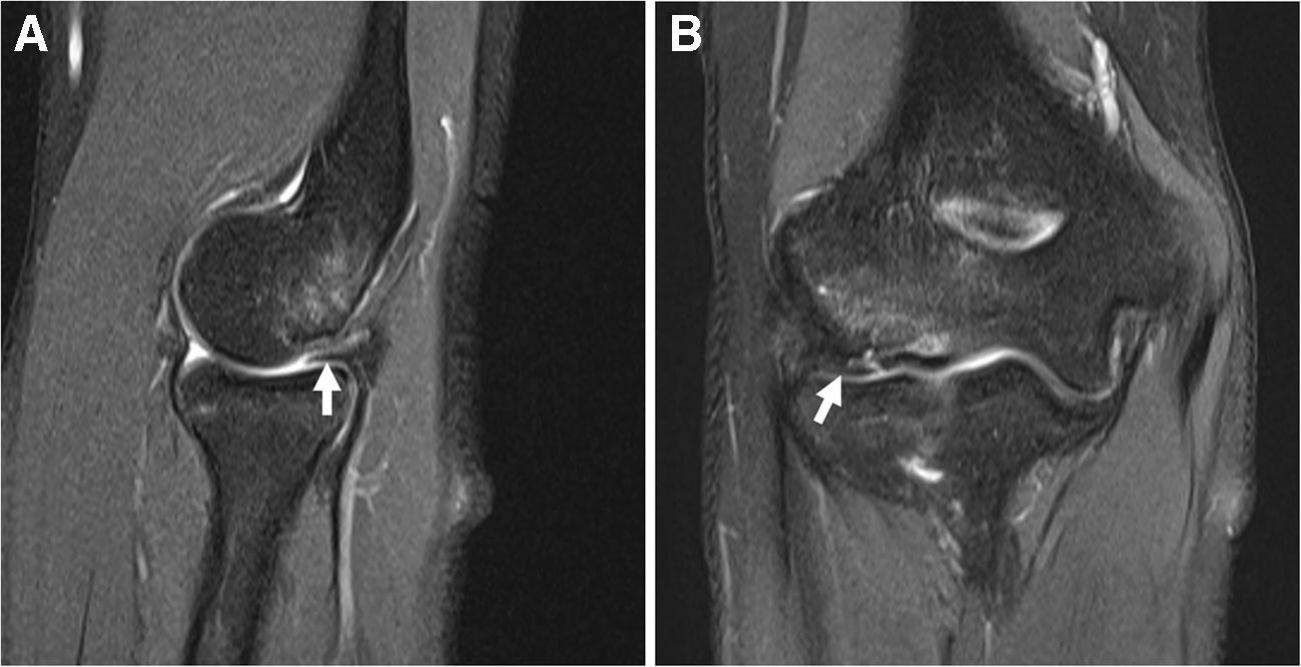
Plica syndrome. Seventeen-year-old elite volleyball player with posterolateral elbow pain. Sagittal (A) and coronal (B) intermediate-weighted fat-suppressed conventional 1.5 T MR images demonstrate a thickened radiocapitellar plica (arrows) with adjacent bone marrow edema, consistent with the clinical diagnosis of impingement. The plica was arthroscopically resected with resolution of symptoms
